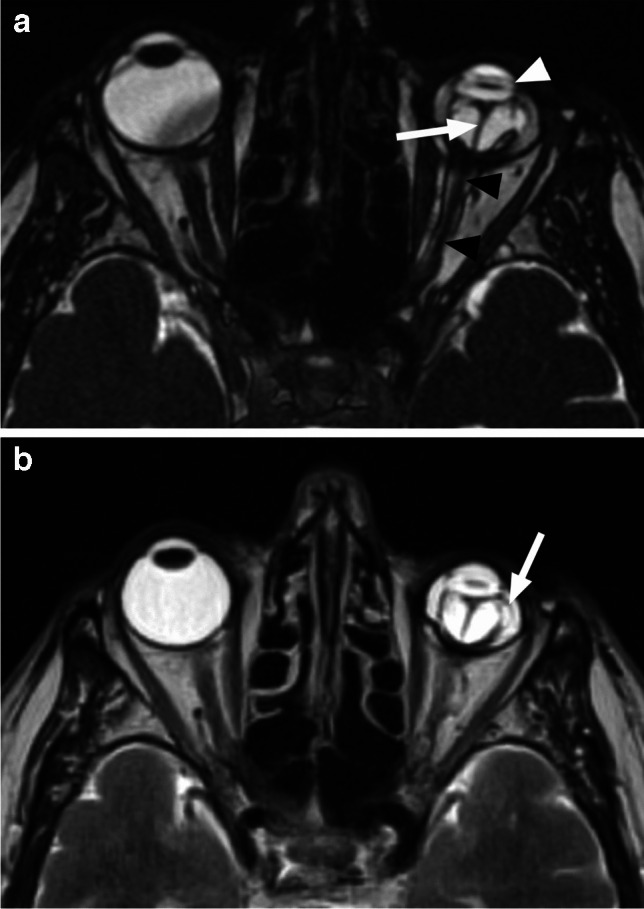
Persistent fetal vasculature (PFV). High resolution 3D T2-weighted image of the orbits in the axial plane (a) demonstrates microphthalmia on the left side with the characteristic central linear band (arrow) along with deformed lens and ciliary body (white arrowhead). There is also small caliber of the optic nerve (black arrowheads), consistent with nerve atrophy. Corresponding axial T2-weighted image (b) shows the same intraocular findings, including a retinal cyst (arrow)
